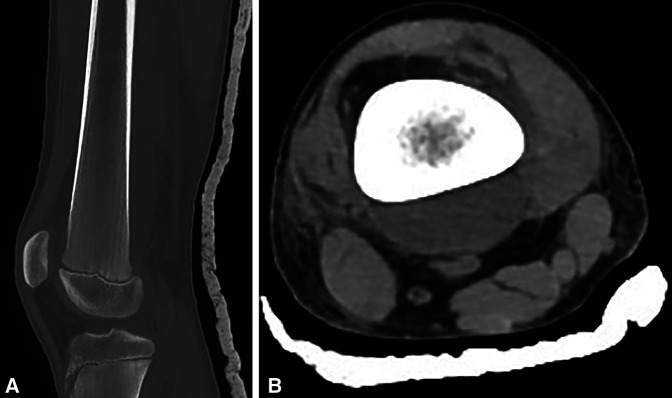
CT scan showing sagittal (A) and transverse (B) views of the distal femur. A medium-density structure in the posterior surface is identified.
